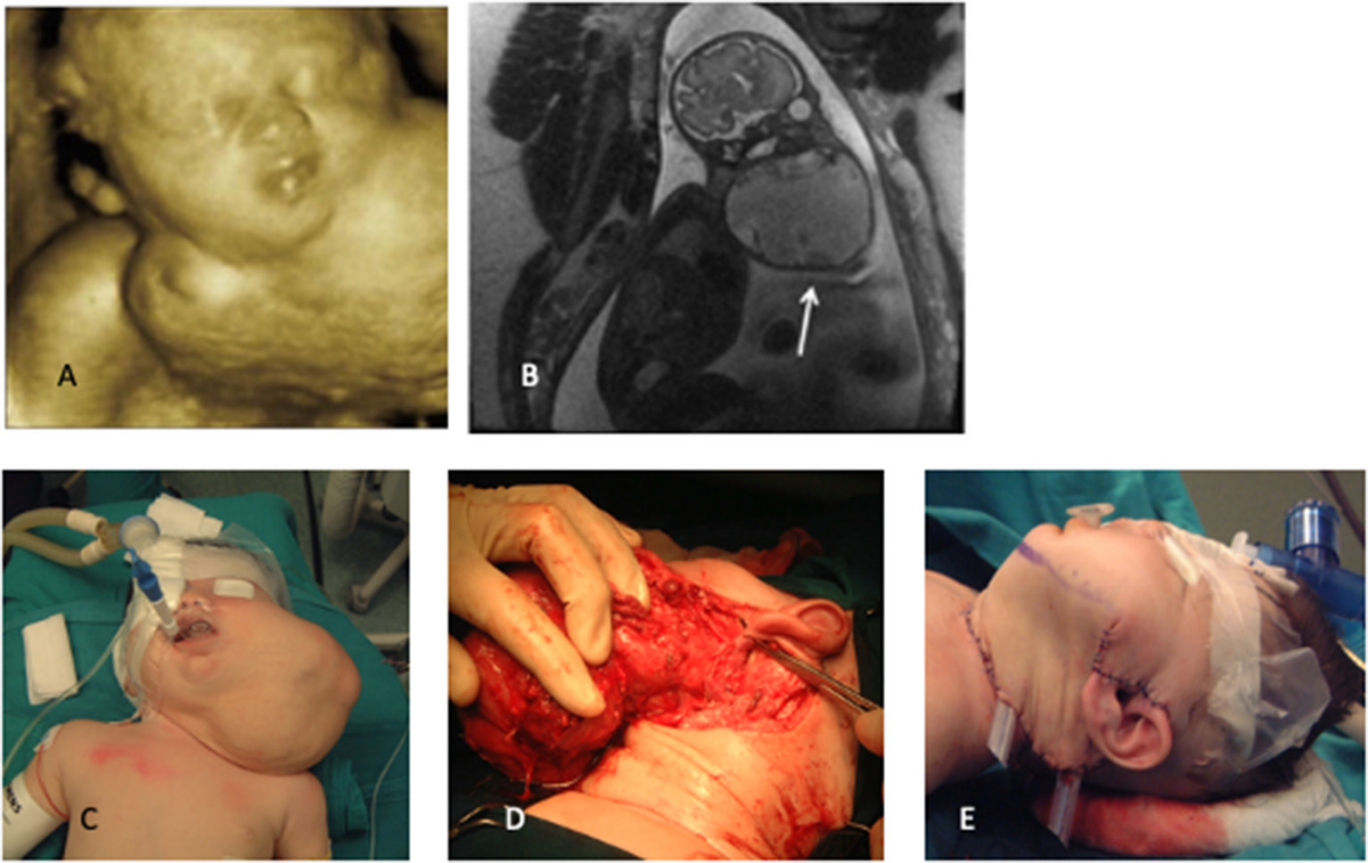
(A) 3D prenatalxc ultrasound, (B) fetal MRI, (C) preoperative appearance, (D) intraoperative dissection, (E) final reconstruction. White arrow indicate LM.
